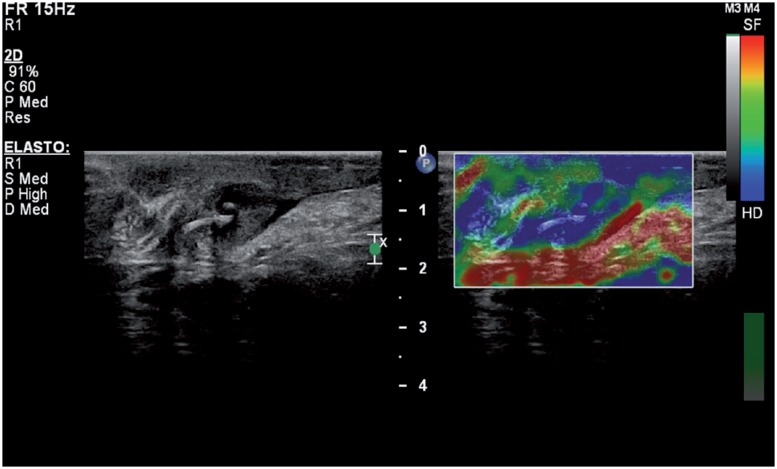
Real time ultrasound elastography demonstrates that the lesion is harder than the surrounding tissues.
